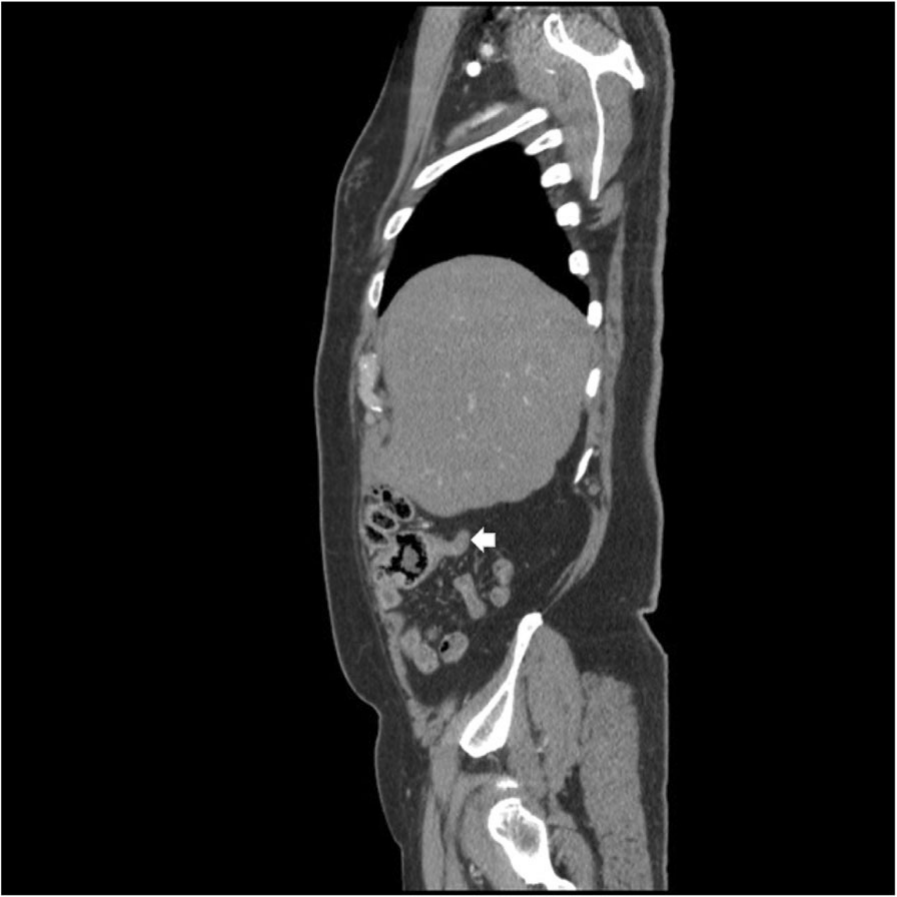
Computed tomography scan of the chest, abdomen, and pelvis showing a thickened appendix at 12 mm in diameter as indicated by *arrow*
